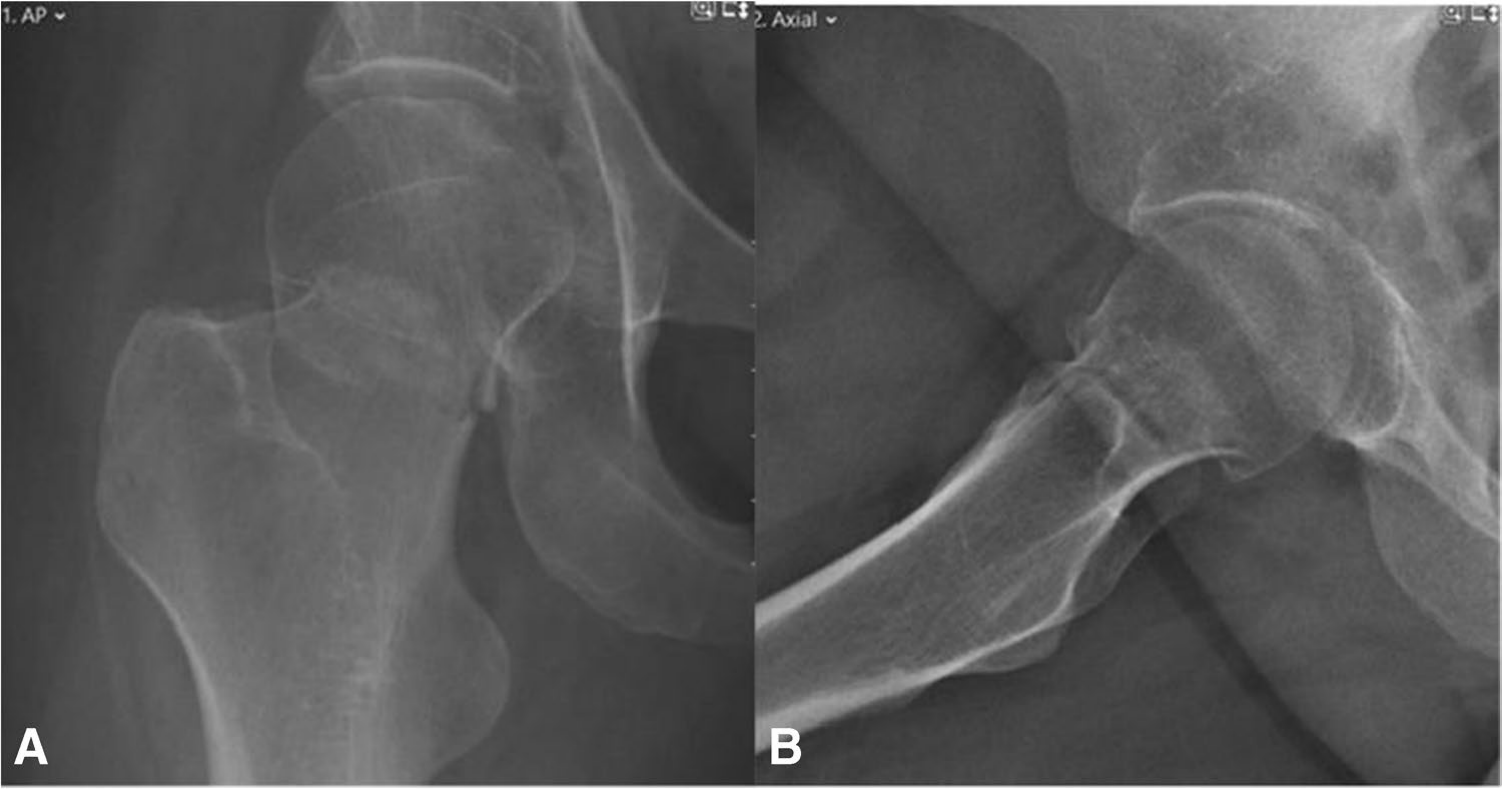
A, B AP and lateral radiographs showing intracapsular femur fracture (displaced, Garden 4) of a 39-year-old female. Past medical history consists of cerebral palsy, walks with a cane. Sustained an isolated injury to the right hip after falling from standing height
